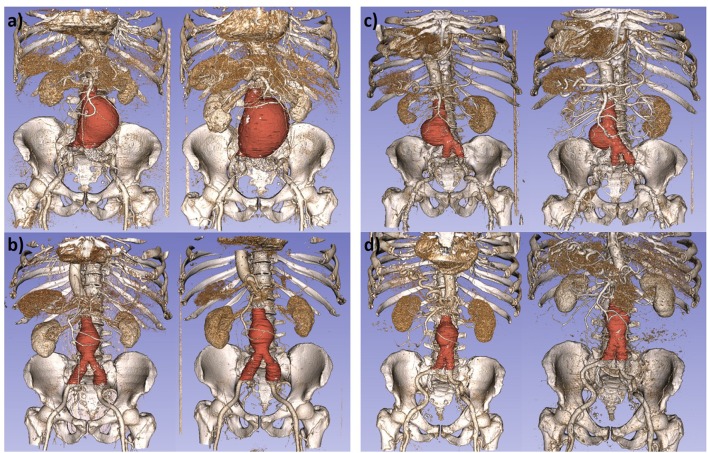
Volume renderings of computed tomography angiography scans, with superimposed aneurysm segmentations for some patients in our study. (a,b) Images of patients with unfavorable evolution at t1 (left) and t2 (right). (c,d) Images of patients with favorable evolution at t1 (left) and t2 (right).
